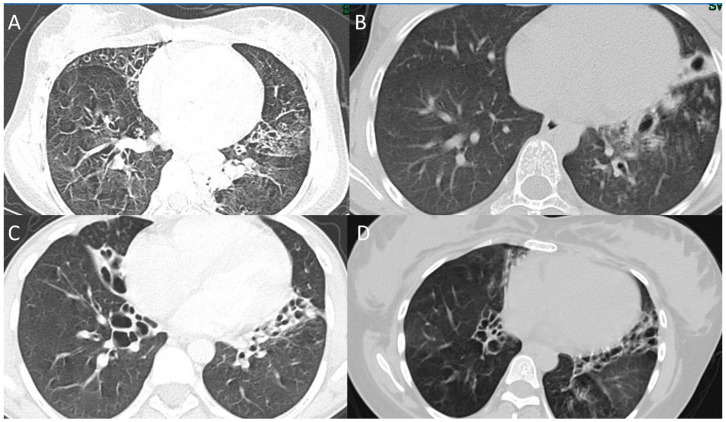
CT scans chest findings (patients 17, 12, 16, and 7). (A) CT scan of patient 17 (14-year-old female with RSPH9 gene mutation) shows mosaics pattern with bilateral bronchiectasis. (B) CT scan of the patient 13 (13-year-old female with TP73 gene mutation) shows left lower lobe cystic bronchiectasis. (C) CT scan of the patient 16 (15-year-old male with DNAH5 gene mutation) shows bilateral cystic bronchiectasis. (D) CT scan of the patient 8 (25-year-old female with DNAH5 gene mutation) shows mosaic attenuation with bilateral bronchiectasis.
